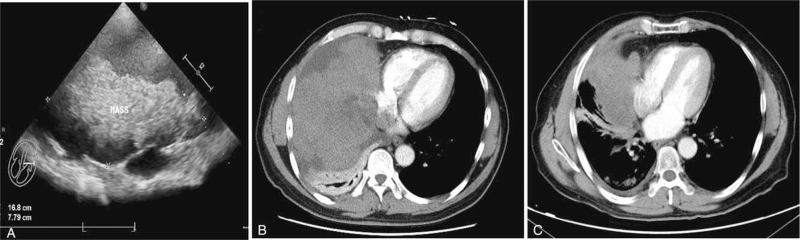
(A) Ultrasonic images: a huge inhomogeneous mass in the right thoracic cavity accompanied invasion of the pericardium. (B) Computed tomography images. A heterogeneous, macro-lobulated mass with inhomogeneous contrast accompanied by partial calcification in the right thorax and mediastinum; (C) After radiotherapy and chemotherapy regimen, the size of the tumor decreased significantly.
